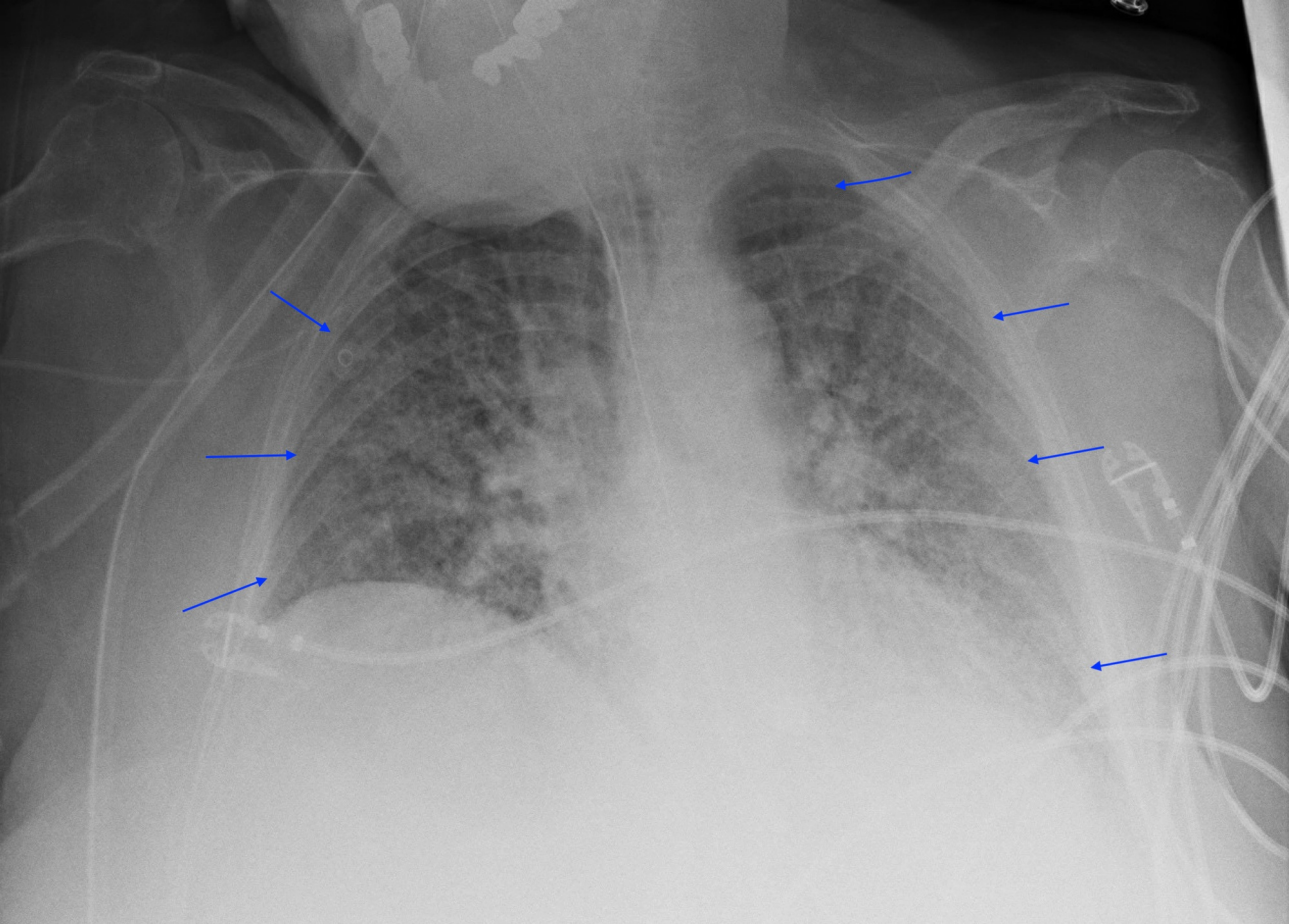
Chest X-Ray Stable diffuse interstitial infiltrates with mild heterogeneous alveolar confluence.
